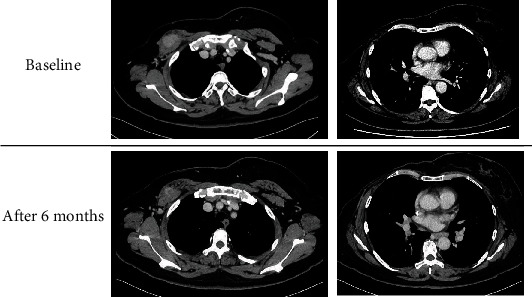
At baseline, computed tomography (CT) shows the appearance of the right subpectoral lymph node metastasis (3.6 cm diameter). After 6 months, CT showed further reduction of that lesion, while the right hilar lymph nodes are enlarged.
